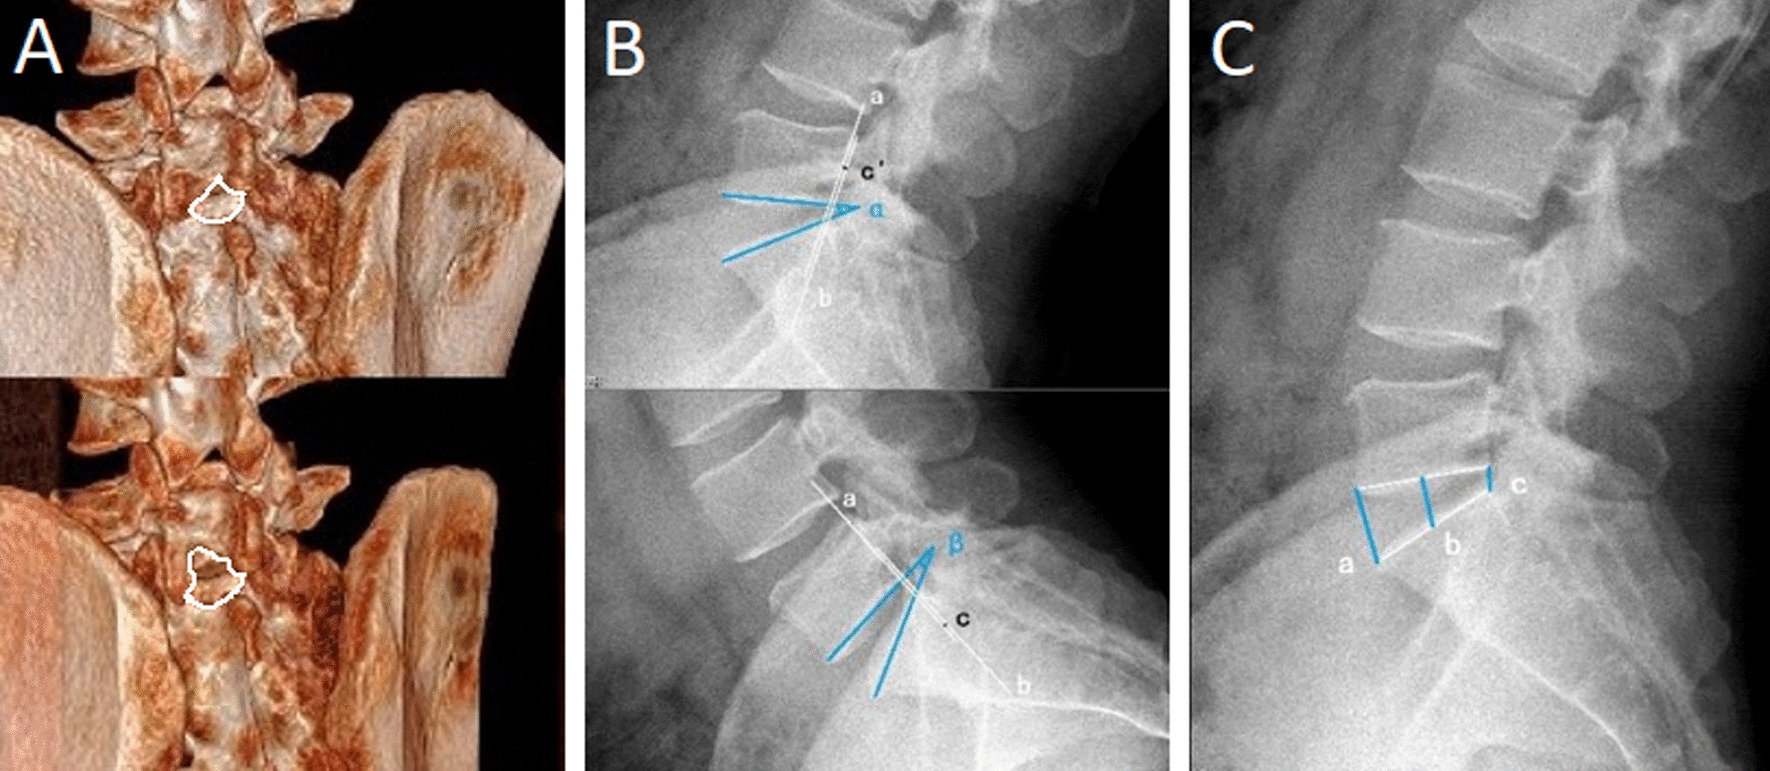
A Measure the area of 3D CT lamina space before and after surgery to estimate the area of bone resection, and BRA = postoperative ISA–preoperative ISA. B Dynamic position X-ray was used to measure ROM and ST before and after surgery, ROM = α − β, ST =|c–c'|. (C) Lateral lumbar X-ray was used to measure DH before and after surgery, DH = (a + b + c)/3
